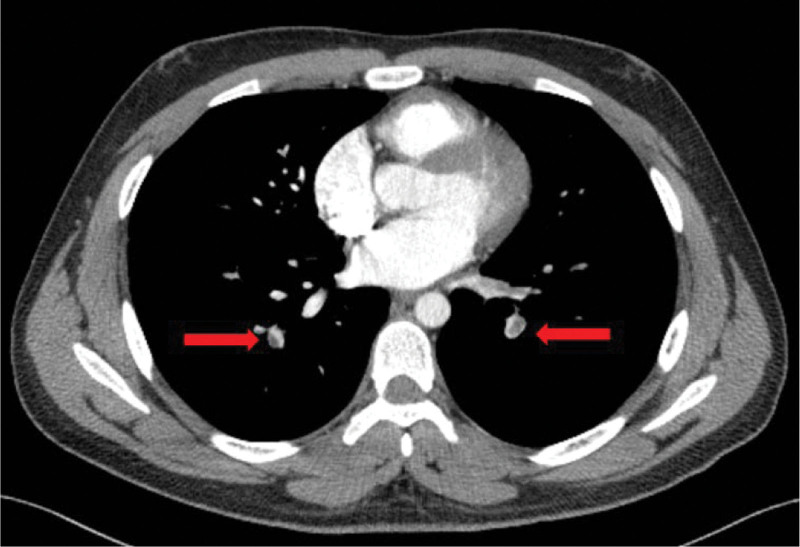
Enhanced chest computed tomography showing pulmonary infarction in the lower lobe of the right lung with multiple embolisms in bilateral pulmonary arteries and branches (red arrow).
